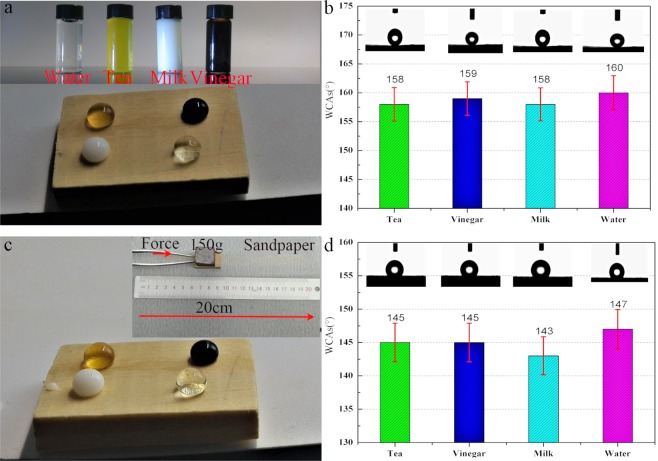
Robust superamphiphobic performances of PVB/SiO_2_/wood measured by (a) an ultrasonic rinse for 24 h and (b) a sand paper abrasion test. (c,d) The corresponding WCAs of the PVB/SiO_2_/wood with liquid droplets of brine, tea, milk and vinegar.
